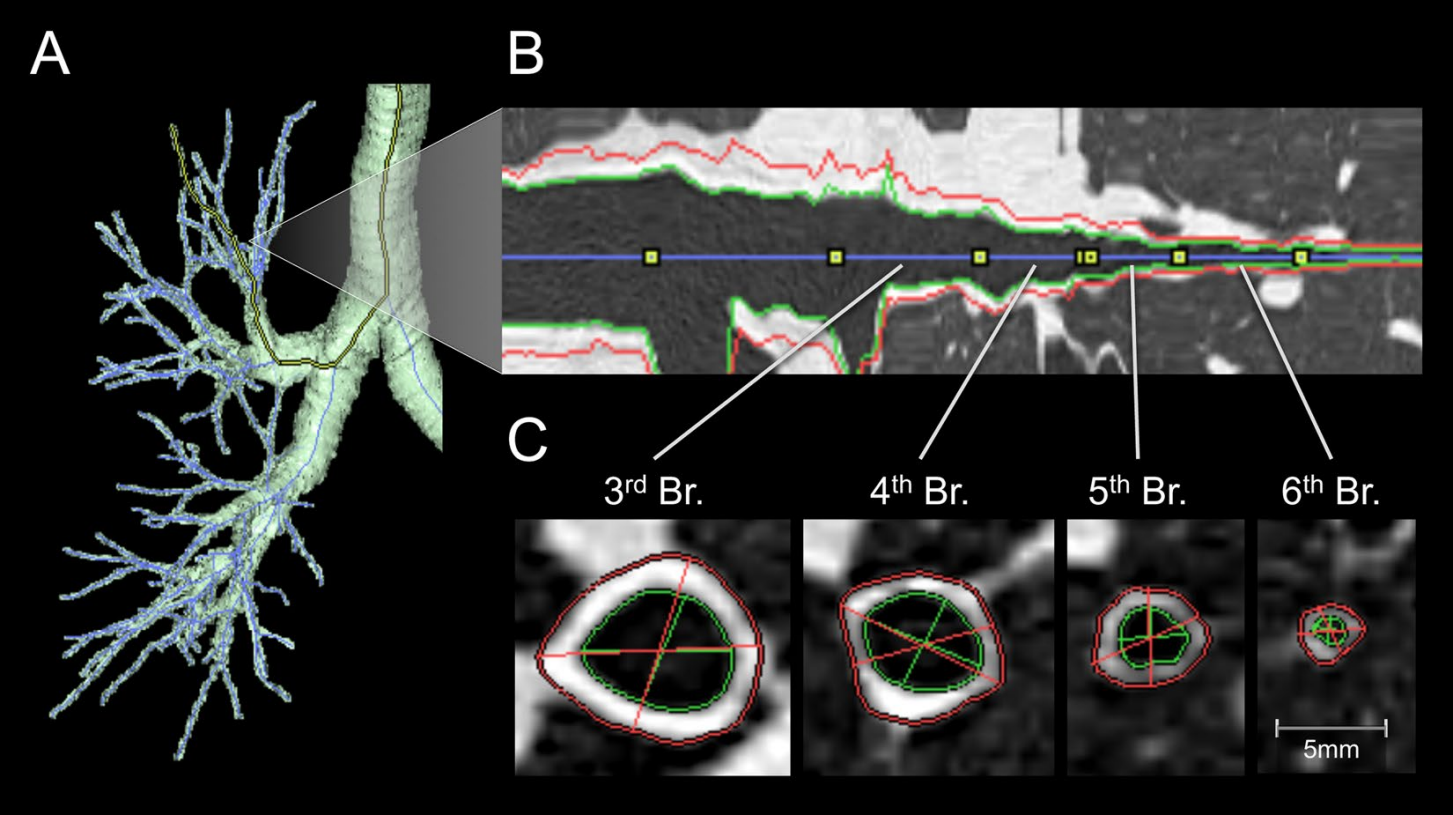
Three-dimensional CT analysis of the airways. (A) Three-dimensional image of the airway trees. (B) Longitudinal image of the reformatted airway. (C) Short axis image of the airway. Red and green lines indicate the outer and inner edges of the airways, respectively. The areas within the green line and between the red and green lines are the airway inner luminal area and wall area, respectively.
